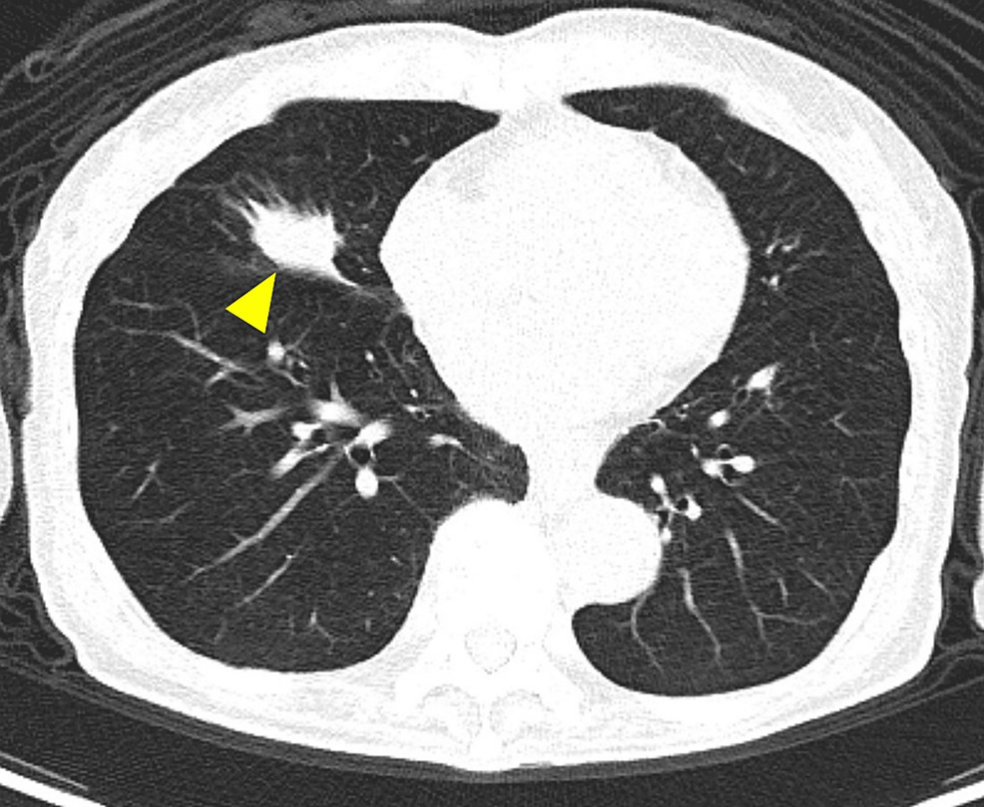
Thoracic computed tomography Thoracic computed tomography shows the irregularly shaped nodule in the middle lobe of the right lung (arrowhead).
